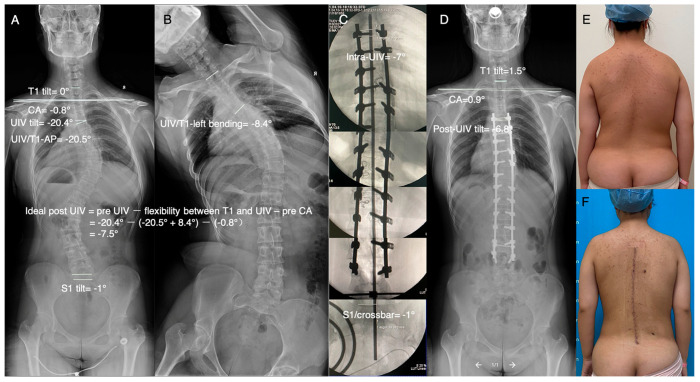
Representative case of using the improved crossbar technique to control the intraoperative UIV tilt to achieve postoperative LSB. (A) AP radiograph of the entire spine preoperatively. (B) Left bending. The ideal postoperative UIV tilt, calculated by the formula using the parameters measured in A and B, was −7.5 degrees. (C) Use of intraoperative fluoroscopic images and the improved crossbar technique to control the intraoperative UIV tilt, which was −7 degrees. (D) AP radiograph of the entire spine postoperatively. The actual postoperative UIV tilt was −6.8 degrees, and the postoperative CA tilt was 0.9 degrees. (E,F) Clinical images of the same patient preoperatively and postoperatively.
